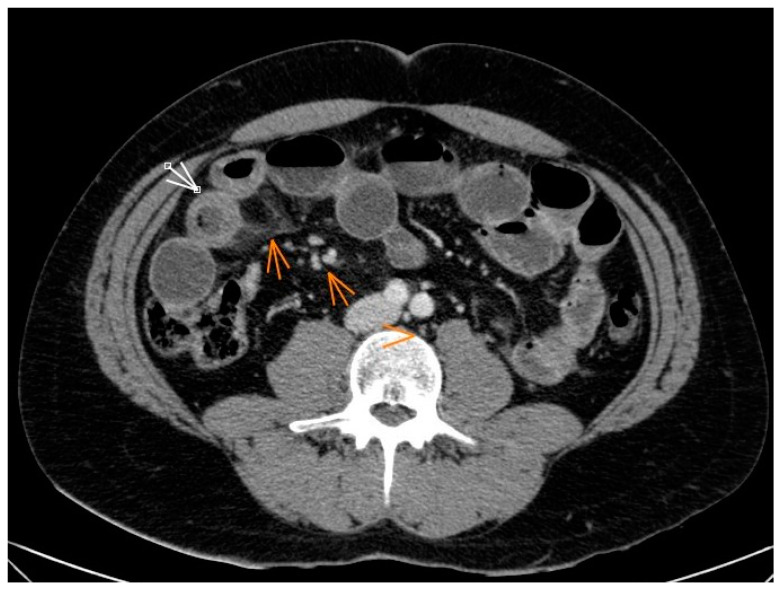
CT abdomen: Thickening of the wall of a short segment of the distal ileum with submucosal edema. Infiltration of mesenteric fat and adjacent subcentimeter lymph nodes. The arrows represent asymmetric mural thickening of the intestinal wall with submucosal edema.
